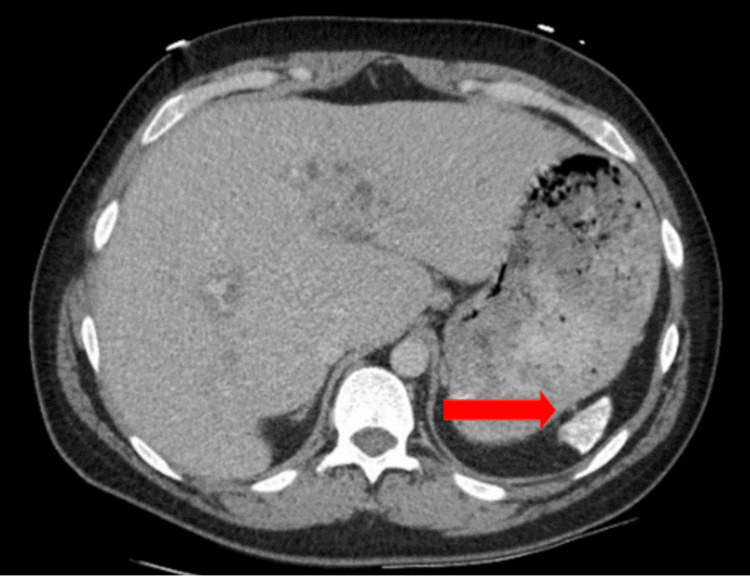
CT abdomen image of hypoplastic spleen. CT: Computed tomography
